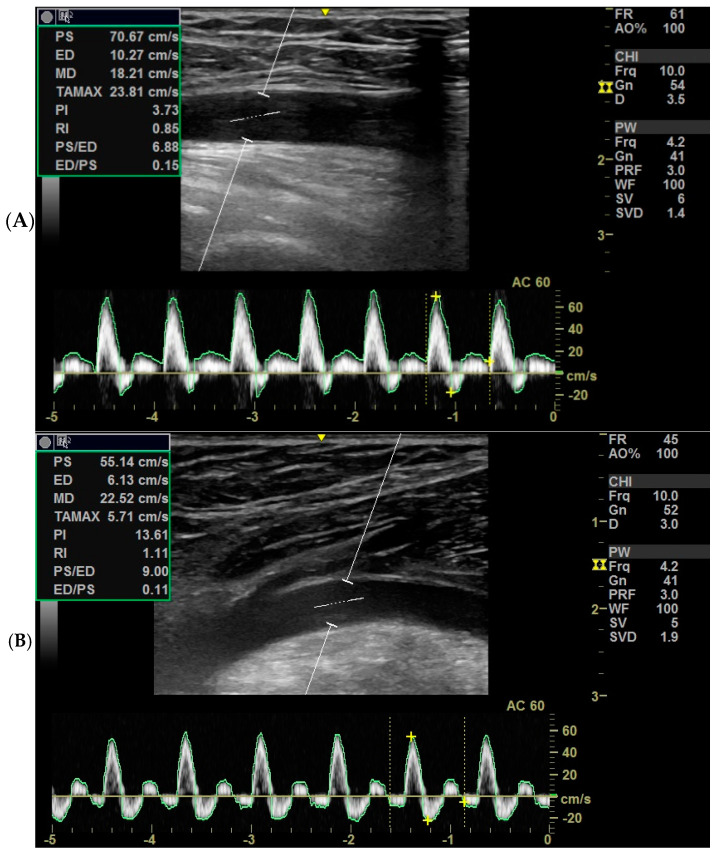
Standard Doppler indices of (A) the femoral artery and (B) the popliteal artery.
